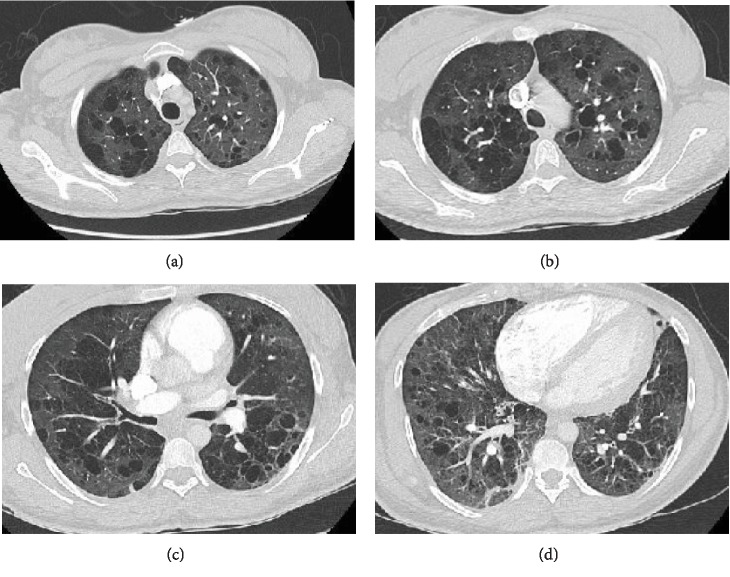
(a–d) CT chest with severe cystic disease involving all lobes with peribronchial thickening and cylindrical bronchiectasis at the bases.
